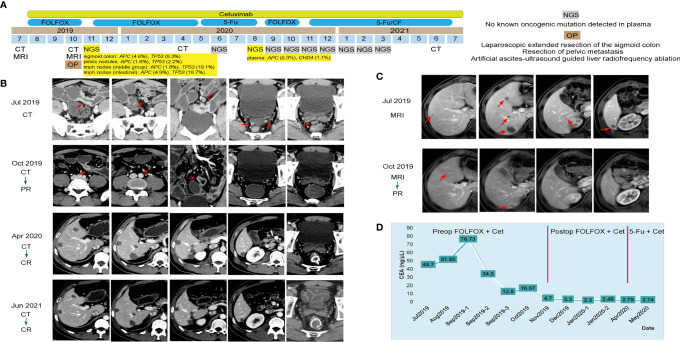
Tumor progression of the patient in case 1. (A) The timeline of diagnosis and treatment. (B) Tumors shrank and disappeared during the course of treatment by computed tomography scans of the patient’s abdomen and pelvis; tumors are indicated by red arrows. (C) Tumors shrank and disappeared during the course of treatment by magnetic resonance imaging of the patient’s abdomen; tumors are indicated by red arrows. (D) Line chart showing the changes in the levels of carcinoembryonic antigen during the course of treatment.
